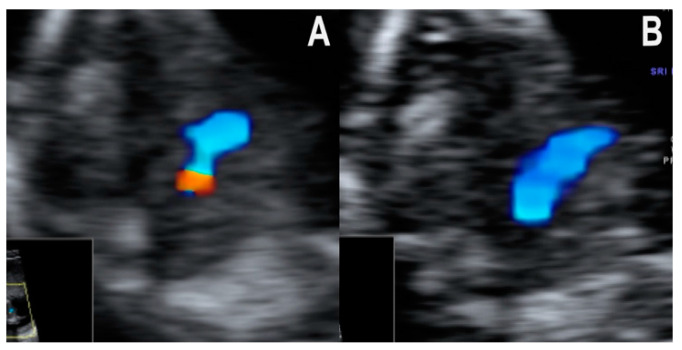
Color doppler flow patterns seen in the three-vessel and trachea view in the case of CAT. (A): Pulmonary arteries arising from the vessel. (B): The straight course of the vessel through the mediastinum.
